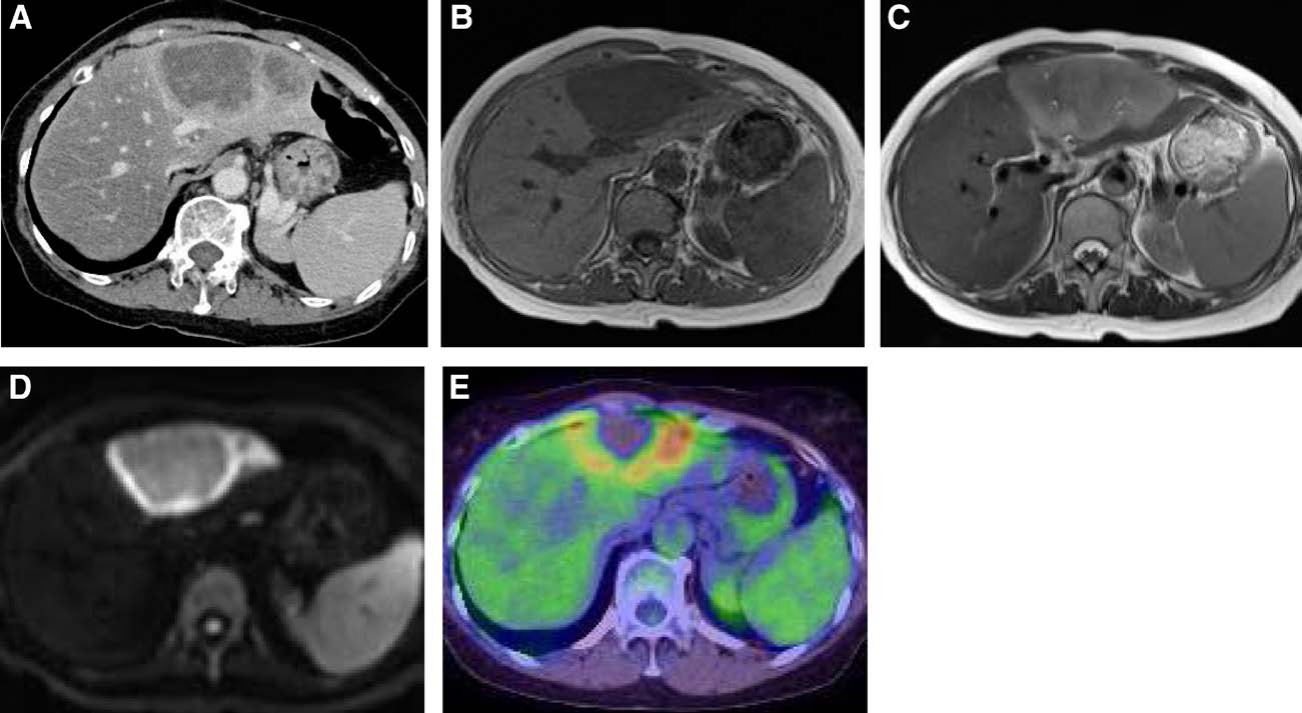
Abdominal contrast-enhanced computed tomography (CT) scan (A), magnetic resonance imaging (MRI) (B, C, D) and 18F-fluorodeoxyglucose positron emission tomography CT (FDG-PEG/CT) (E) in the present patient. Enhanced CT scan in the late phase shows annular enhancement at the tumor edge and a low-density area in the center of the tumor (A). MRI showed low intensity of the tumor on T1-weighted images (B), faint high intensity on T2-weighted images (C), and high intensity at the tumor edge on diffusion images (D). FDG-PEG/CT scan images showed abnormal accumulation of FDG mainly at the tumor edge in the lateral segment [maximum standardized uptake value, standardized uptake value max (SUVmax) = 8.22] (E). CT = computed tomography, FDG-PEG = fluorodeoxyglucose positron emission tomography, MRI = magnetic resonance imaging.
